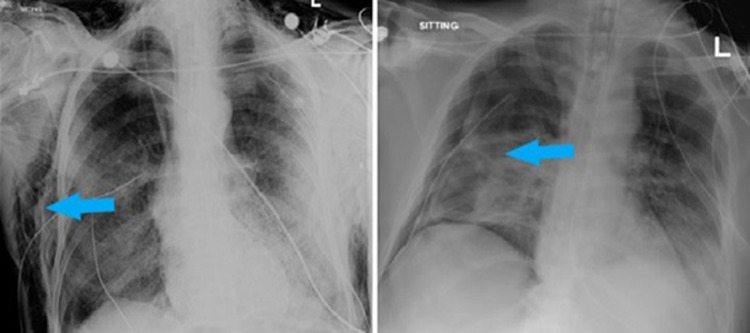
Chest x-ray showing SE (right image) and pneumothorax with chest tube on right side (left image).
